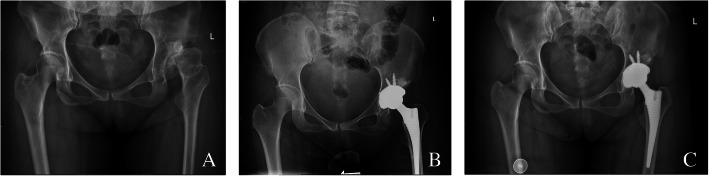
a Patient 17, severe DDH on the left side. b X-rays after operation for 3 days. c review after 3 year, the position of the component is stable
